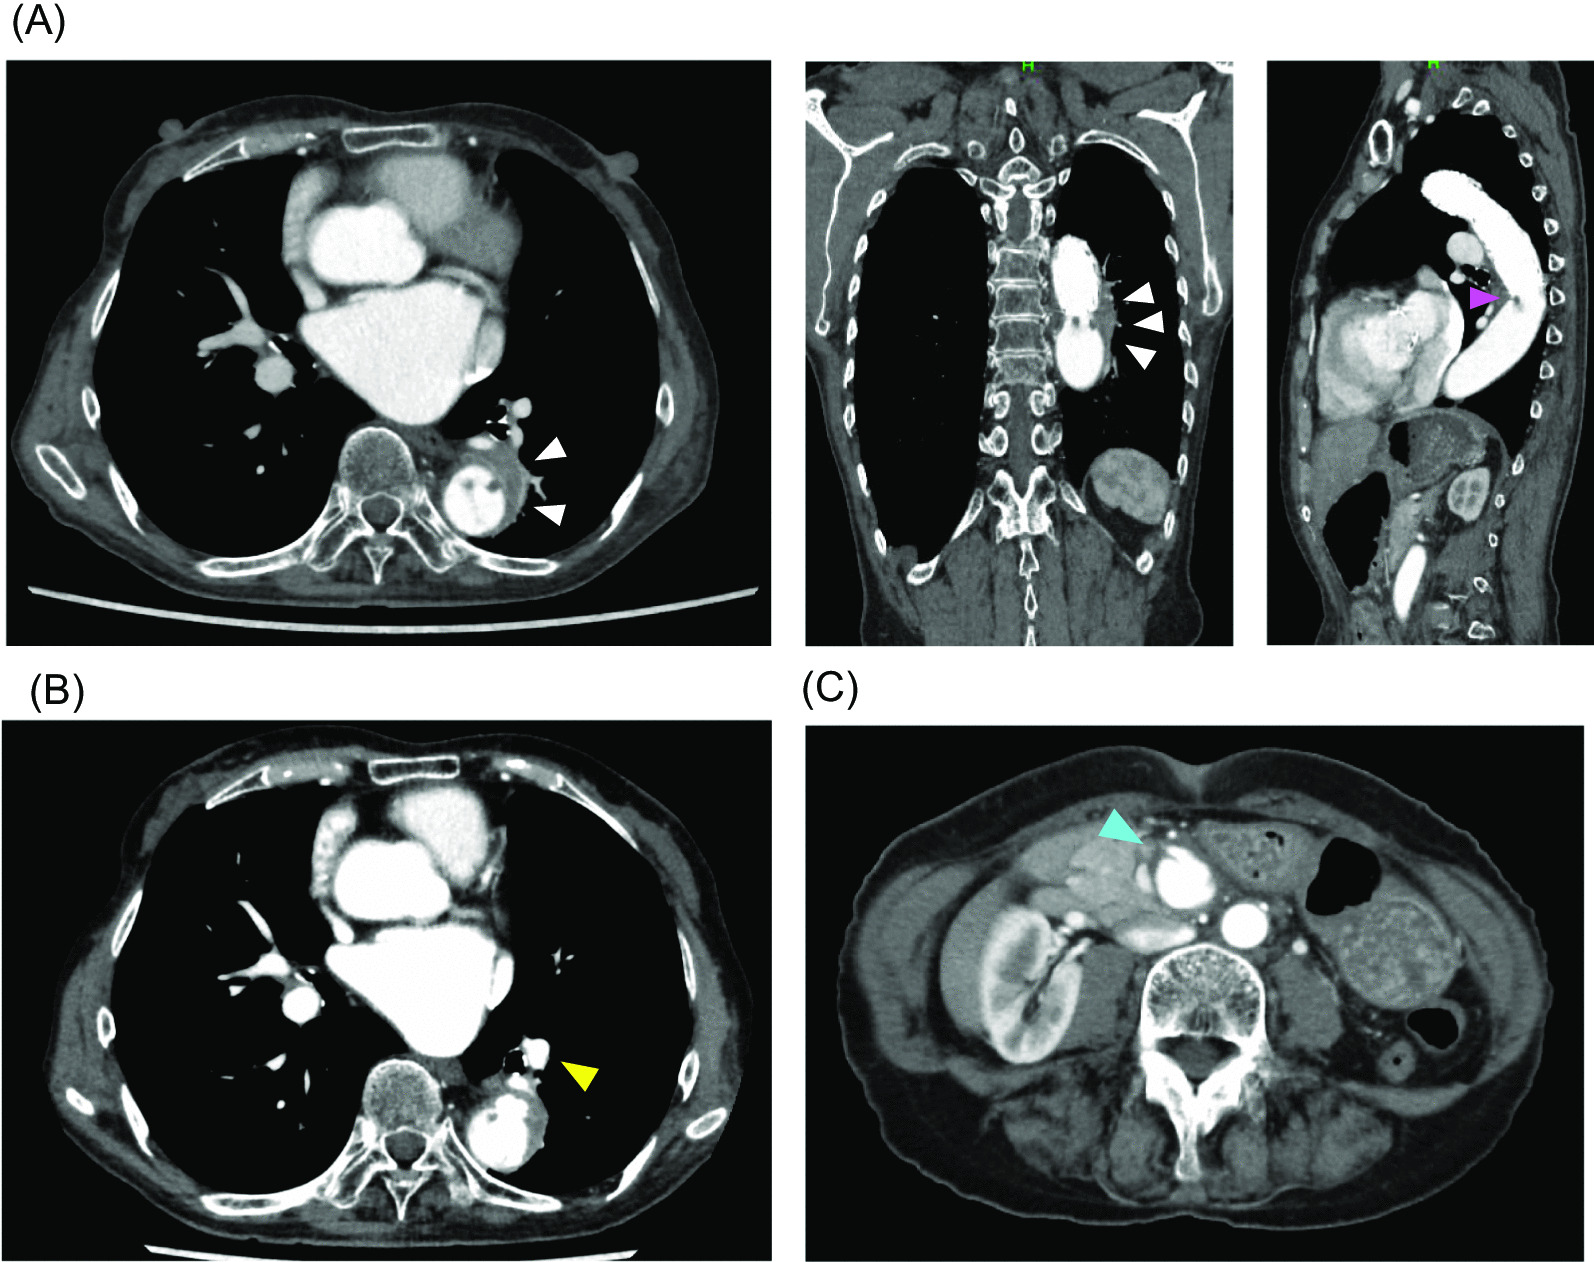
The thoracic and abdominal enhanced-CT in the second admission due to hemoptysis. A A pseudo aneurysm surrounding the distal end of the descending aortic graft (white arrows). The areas without CT-enhancement in the graft lumen indicate thrombus formation. A magenta arrow indicates the bent junction of the descending graft and aorta. B Formation of the fistula from the pseudo aneurysm to pulmonary vein and trachea (yellow arrow). C Enlargement of pseudo aneurysm at the obstructed part of the SMA (cyan arrow)
